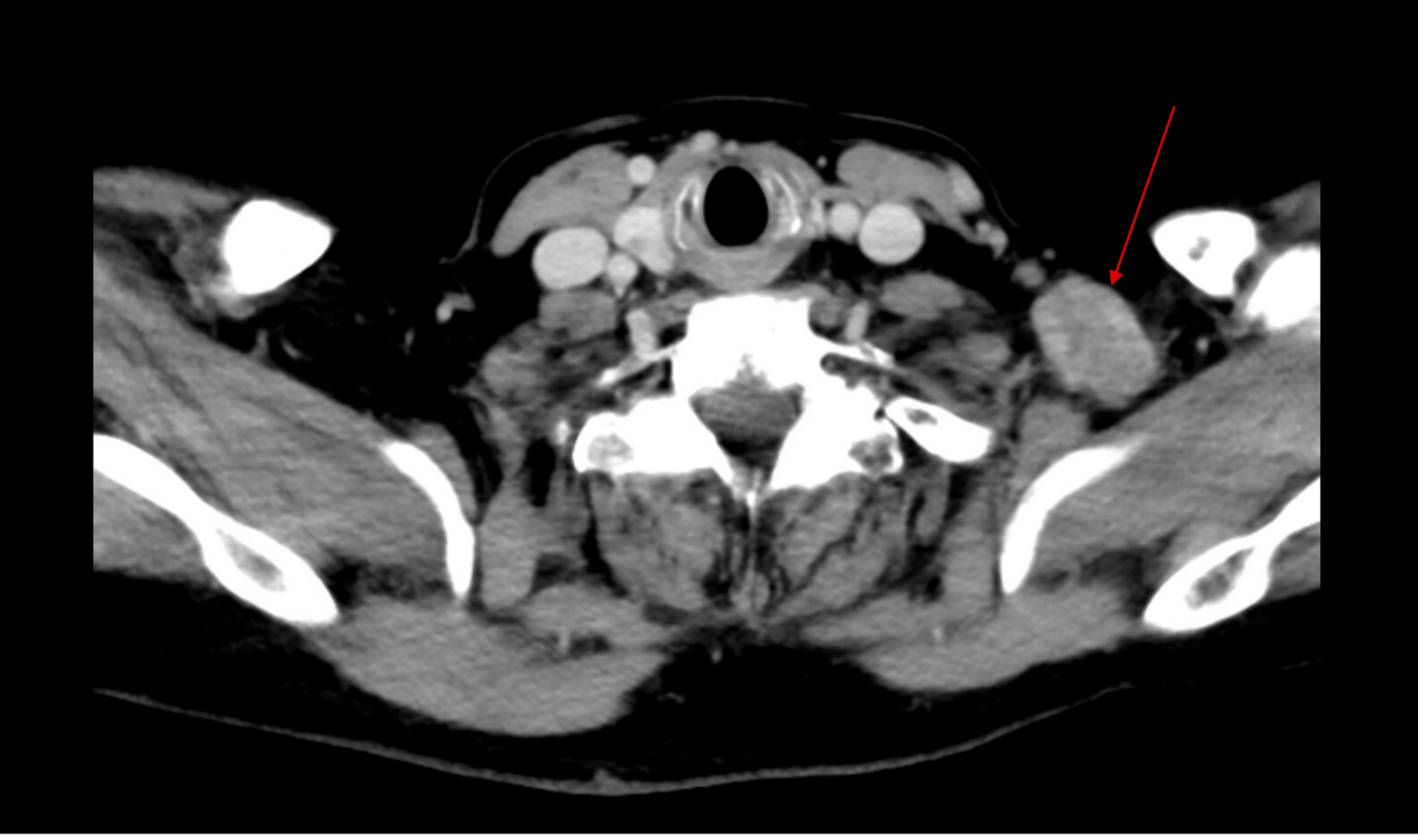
Axial view of a CT soft tissue neck with contrast, highlighting an enlarged left supraclavicular lymph node indicated by a red arrow. CT: computed tomography
